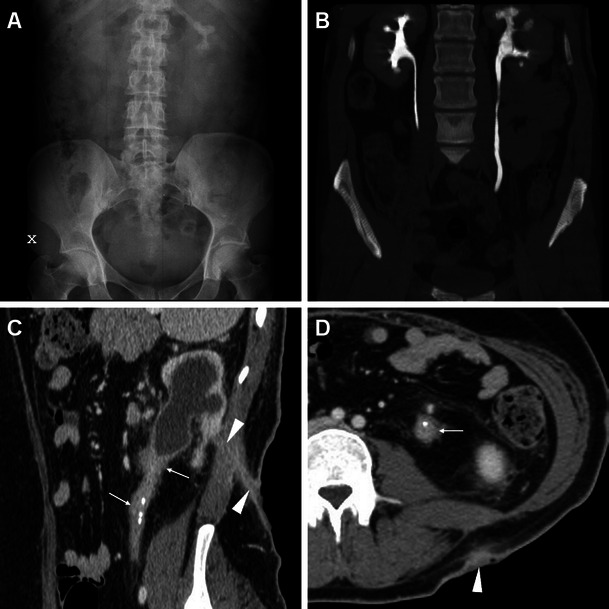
A 45-year-old female with diabetes, antiretroviral-treated human immunodeficiency virus (HIV) infection and left partial staghorn nephrolithiasis at previous plain radiograph (A) and MDCT urography (B) presented 9 weeks after percutaneous nephrolithotomy (PCNL) with clinical and contrast-enhanced MDCT (C, D) evidence of nephrocutaneous fistulisation reaching a cutaneous orifice (*arrowheads*). Associated findings included ipsilateral hydronephrosis with parenchymal thinning, severely thickened hyperenhancing urothelium (*thin arrows*) in the ureteropelvic junction, proximal and mid-ureter, and multiple residual stone fragments along the lumbar ureter consistent with steinstrasse. Surgical treatment included nephro-ureterectomy with fistula debridement. (Partly reprinted with permission from [51])
